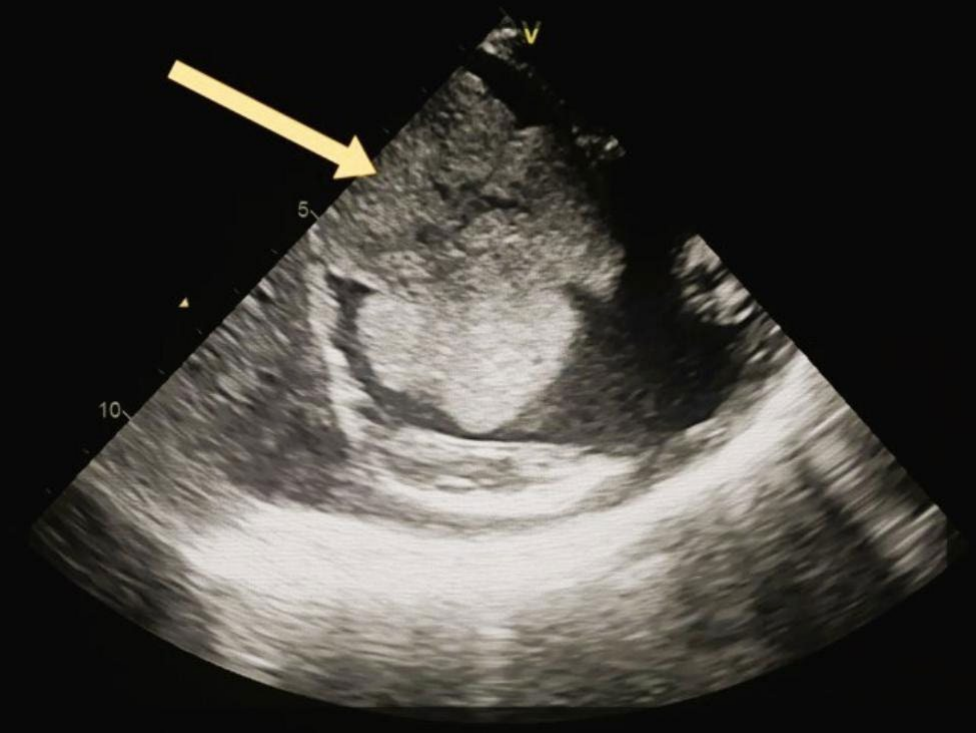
A bicaval view by trans-thoracic echocardiogram which represents a cauliflower mass in the right atrium with a pedicle in the middle, which raised suspicion of myxoma covered with a thrombus
